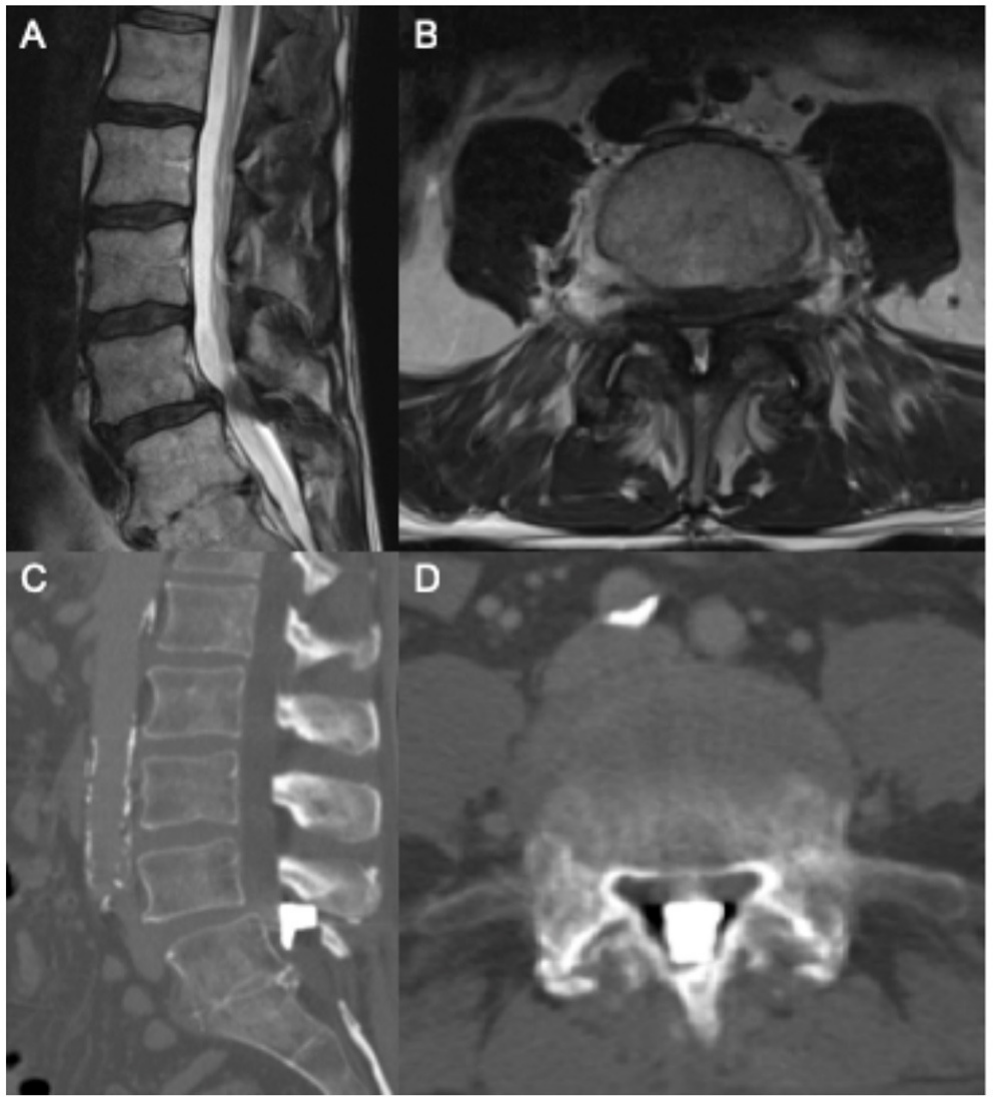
Pre-operative sagittal (A) and axial (B) T2 MRI showing L4/5 severe central canal stenosis. Following interspinous spacer placement, sagittal (C) and axial (D) CT scan showing spacer migration into central canal.
